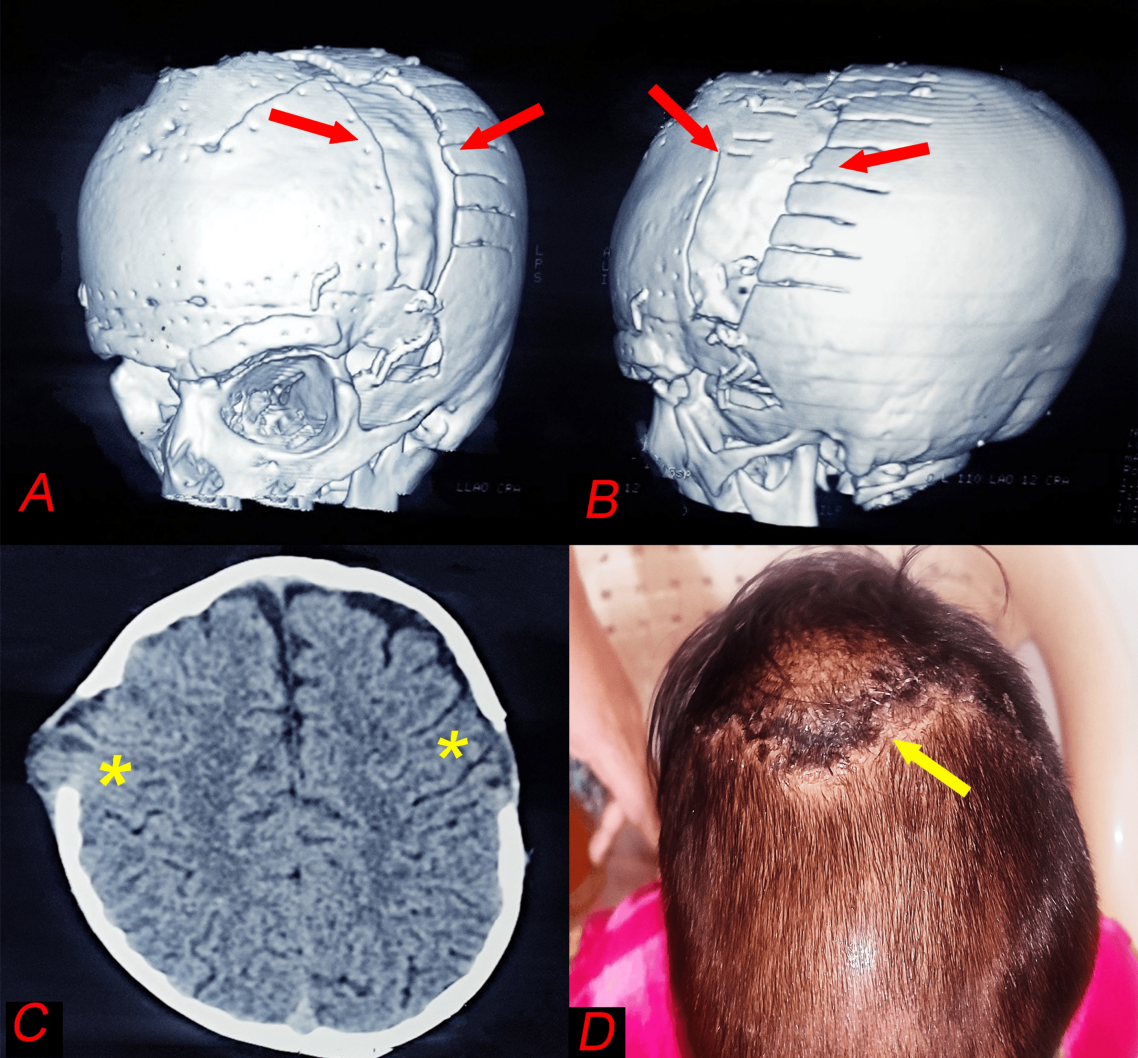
3D reconstructed CT images of the skull following frontal orbital advancement and cranial vault remodeling (red arrows), showing the expanded anterior and posterior cranial vaults with evidence of surgical remodeling and stabilisation (A and B), axial CT image displaying the intracranial view of the cranial vault after surgical intervention, demonstrating craniotomy opening (asterisks) (C), and clinical photograph of the postoperative head showing the healed surgical site with evidence of scalp integrity restoration (yellow arrow) and no significant external deformity (D).
